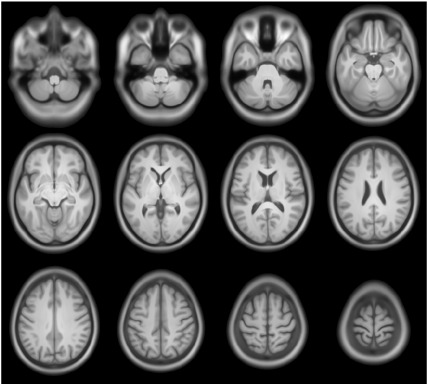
Average of 450 T1-weighted scans from the IXI dataset, which have been aligned using a geodesic shooting model. The left side of the brain is shown towards the left of the image.
